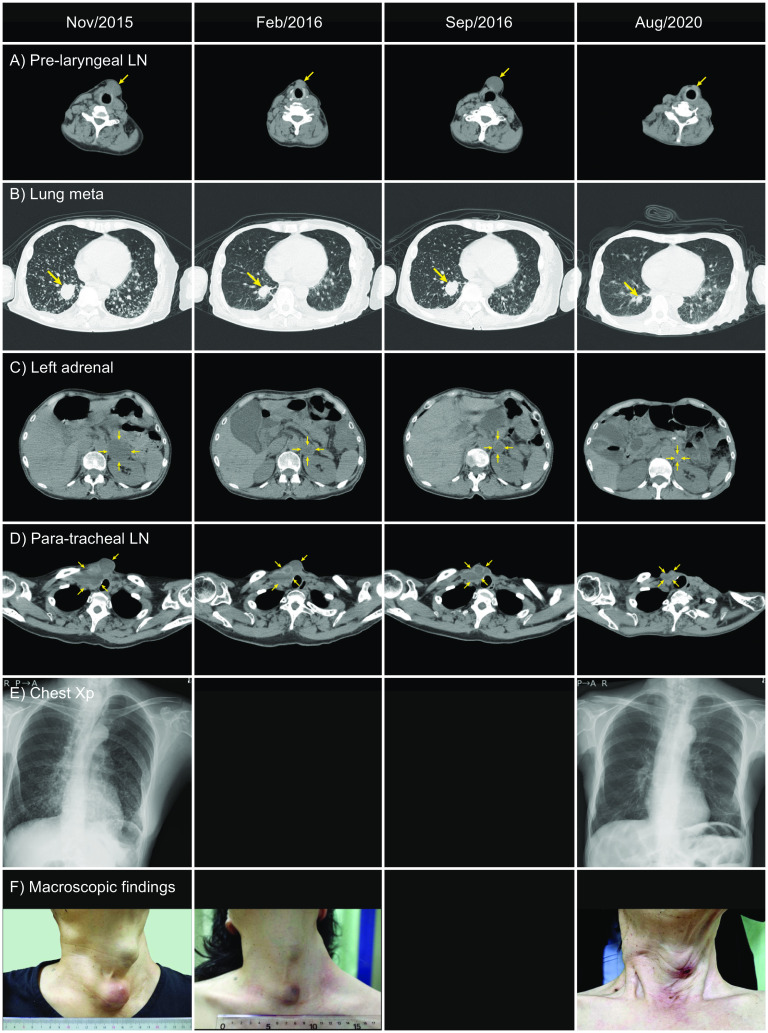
Changes in images from computed tomography (CT) scans (A–D) and chest X-rays (Xp) (E), and macroscopic appearances of the patient’s neck (F) during her clinical course. Enlarged pre-laryngeal lymph node (LN), lung metastasis, left adrenal metastasis, and cystic lymph nodes in the neck and mediastinum are shown in A–D, respectively
